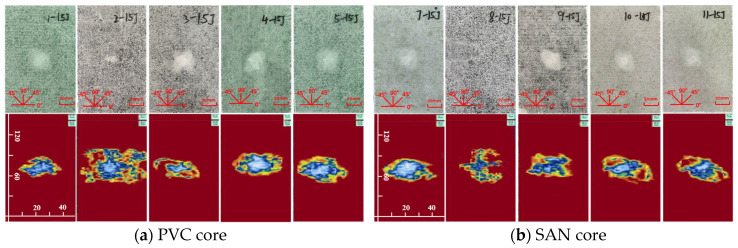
Physical picture of 15 J impact damage and ultrasonic C-scan image.
